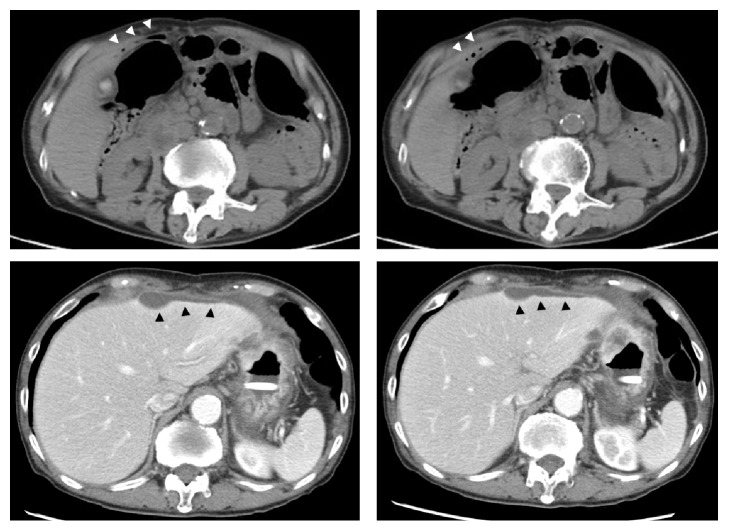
Computed tomography of abdomen and pelvis, showing abnormal pneumoperitoneum (white arrowhead) and limited ascites (black arrowhead).
